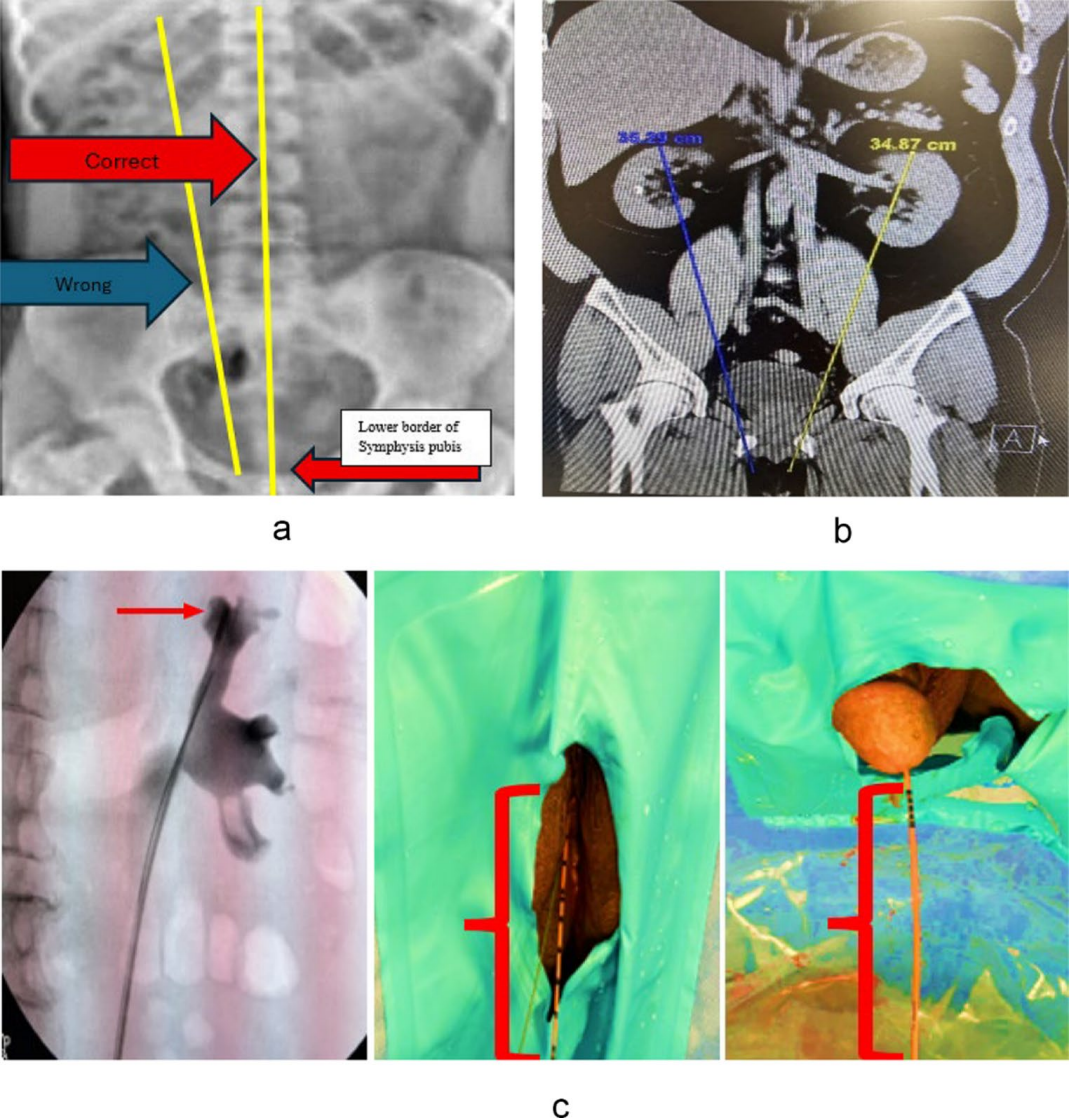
a Subjective measurement on X-ray KUB from T12 to pubic symphysis. b Objective measurement on CT KUB coronal view from the most convex point of the upper pole of the kidney to pubic symphysis: Choose a Coronal view of the KUB that must include upper border of the most convex point of the upper pole of the kidney. Then draw a line towards the lower border of the pubic rami as shown. c Real-time dynamic measurement from the upper pole to urethral meatus from on-table retrograde pyelogram (RPG) via ureteric catheter placed all the way to the uppermost part of upper pole and externally 5 cm beyond the meatus
